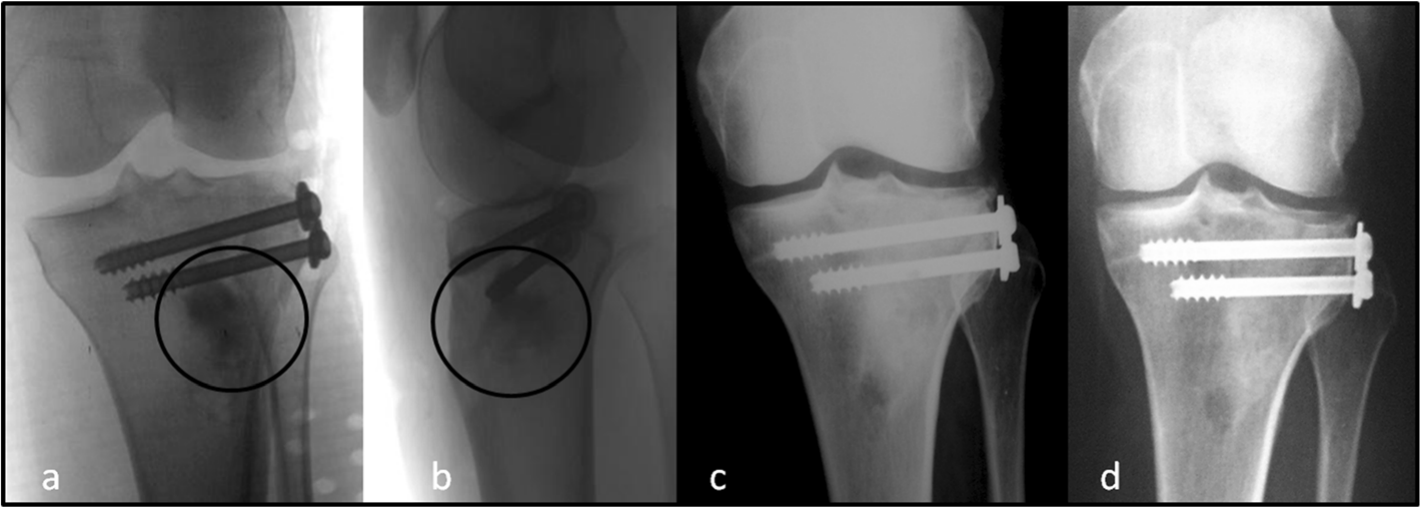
X-rays follow-up at different steps. (a, b) The positioning of the bioceramic may been seen on the immediate post-operative frontal and sagittal x-rays; (c) On the same views at 1 month post-op there is no further evidence of radiocontrast of the bone cement indicating the washout of iohexol; (d) The remodeling process is now progressing. New bone appears well consolidated at 3 months
